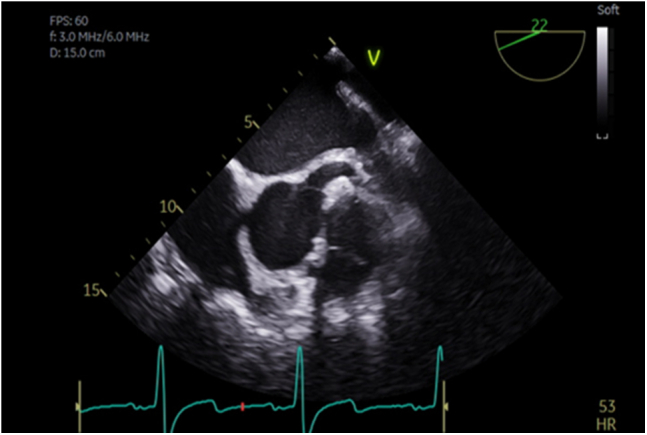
Echocardiogram picture of right coronary artery origin and course.
